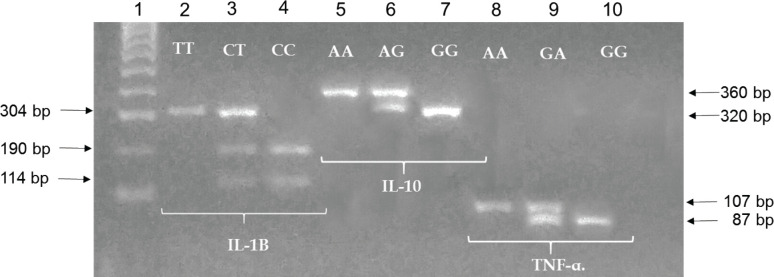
Electrophoretic pattern of the digestion products by RFLP of the polymorphic regions -511, -1082 and -308, IL1-B, IL-10 and TNF-A genes, respectively. 1.5% agarose gel electrophoresis. Lane 1: molecular marker (100 bp DNA ladder); lanes 2–4: the arrows indicate the 304, 190 and 114 bp bands of the digestion products obtained with AvaI enzyme, corresponding to the mutant homozygous genotypes (TT), heterozygous (CT) and homozygous (CC) of the -511 region of IL1-B gene. Lanes 5–7: the arrows indicate the 360 and 320 bp bands produced by digestion with BseRI restriction enzyme, which correspond to homozygous genotype (AA), heterozygous (AA) and homozygous (GG), -1082 region of IL-10 gene. Lanes 8–10: the arrows indicate the 107 and 87 bp band products of the digestion with NcoI that correspond to the AA mutant, GA heterozygous and GG homozygous normal genotypes, -308 region of the TNF-A gene.
